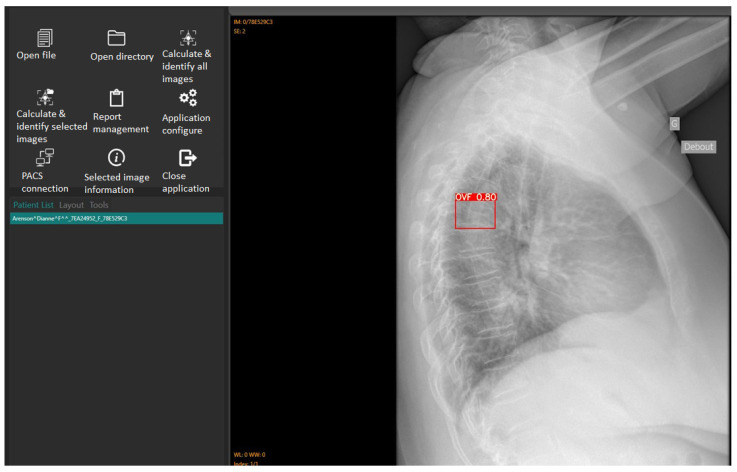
The interface of the Ofeye 1.0 AI tool showing the steps from loading DICOM images to calculating and identifying OVFs on the lateral chest radiographs. In this case, there is a likelihood of an 80% risk of having a fracture.
